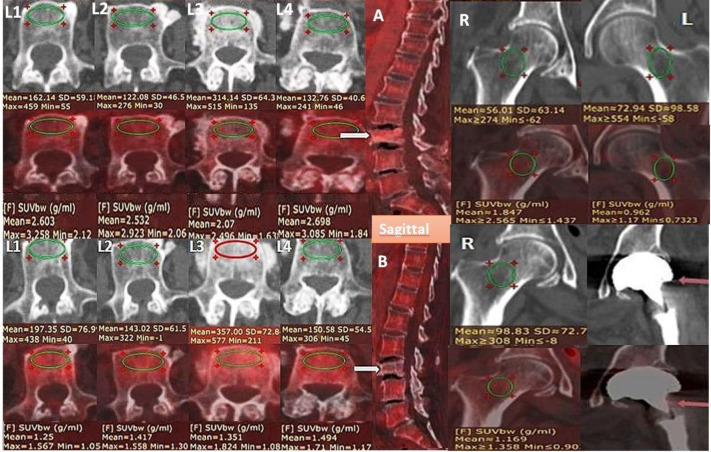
Initial staging (top rows) and post treatment axial PET/CT images (bottom rows) in a 66 year old female. Pretreatment CECT (top row) shows reduced VHU of L1, L2 and L4 with increase attenuation and sclerosis at L3. Sagittal images (A and B) shows compression/collapse of L3 vertebra (white arrow). (R and L in top rows) also show reduced bilateral FNHU. L1-L4 mean HU is 182.7 which is erronously high because of abnormal false high attenuation value caused by collapsed L3 vertebra, while mean L1-L4 SUV_max_ is 2.91; Mean FNHU and mean SUV are 64.5 HU and 1.86 respectively). Post treatment follow up PET/CT images (Bottom rows) 8 months later shows increase in attenuation of corresponding vertebrae with minimally reduced SUV_max_ values (L1-L4 mean HU ~ 212; and mean SUV_ma_x 1.66; an overall increase of ~11% in mean VHU and ~10% decrease in mean SUV_max_; whereas right femoral neck CT attenuation got increased by 75% and SUV_max_ declined by 47% respectively). In addition, on follow up scan, fracture of left femoral neck was also noted with left unipolar hemiarthroplasty. This indicates that conventional bisphophonates may not be very effective in preventing osteoporotic fractures and therefore newer drugs like RANKL inhibitor human monoclonal antibody Denosumab which has shown superior results in prevention of osteoporotic fractures may be preferred in severe osteoporosis. Comparative DXA scans were not available
